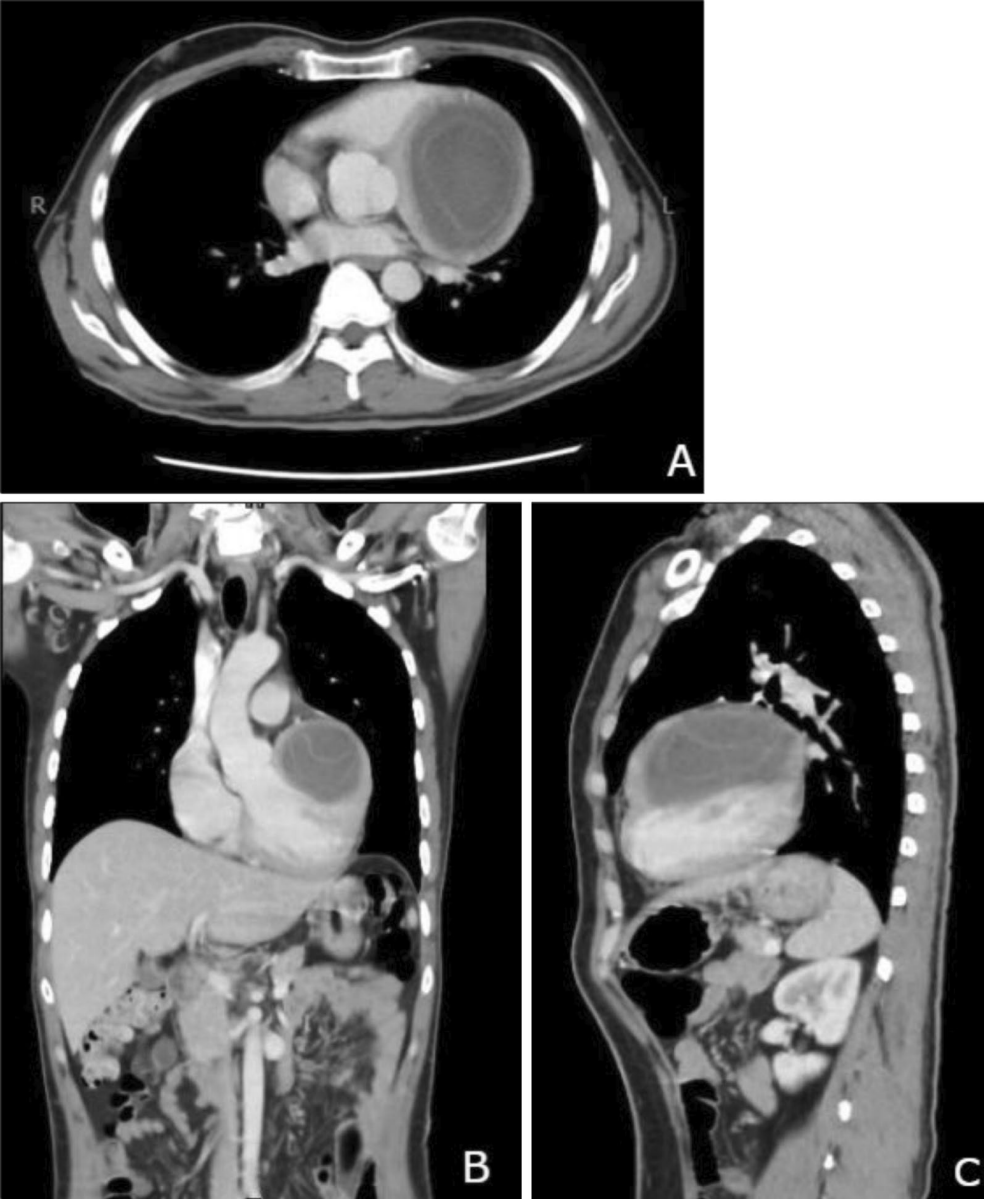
CT Chest shows a large well-defined cystic lesion with multiple internal detached membranes compressing the left ventricle. A through C (Axial, Coronal and Sagittal views)
